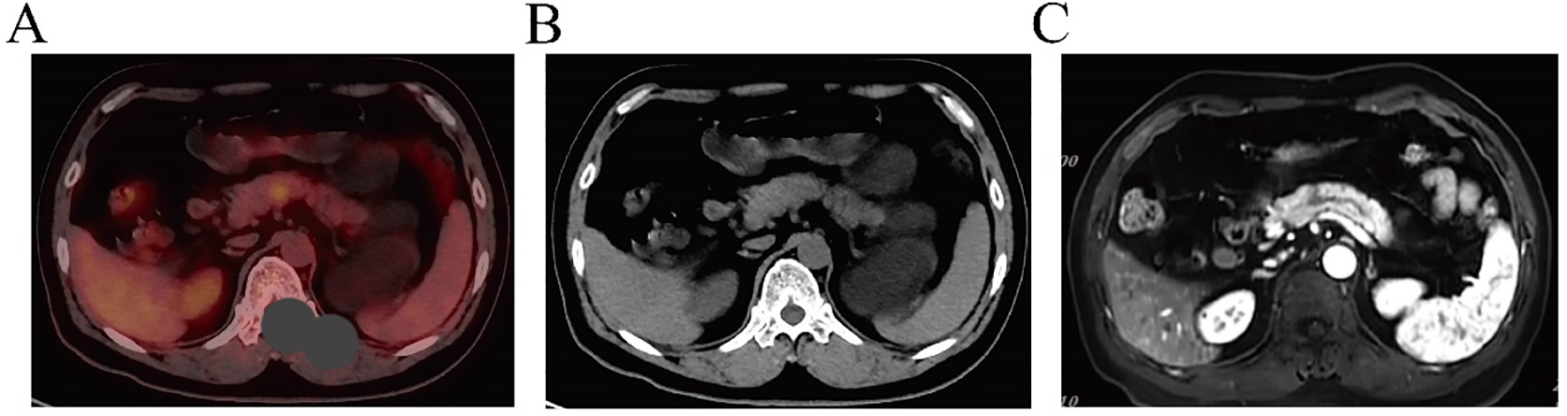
(A, B) PET/CT indicates pancreatic lesion as a metastatic tumor. (C) MRI features suggest that the pancreatic lesion is consistent with metastasis.
